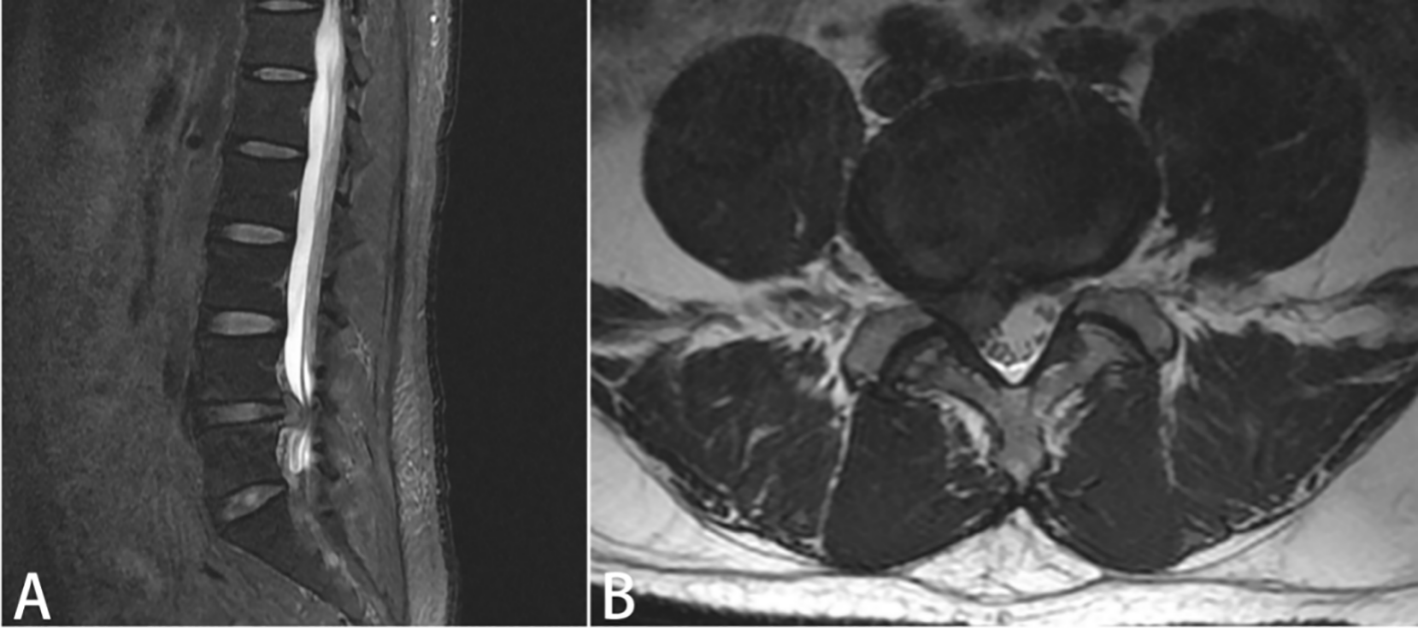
The patient’s preoperative lumbar MRI (A): Sagittal view, (B): Coronal view) reveals a right paracentral herniation at the L4/5 intervertebral disc, with compression of the right nerve root.
